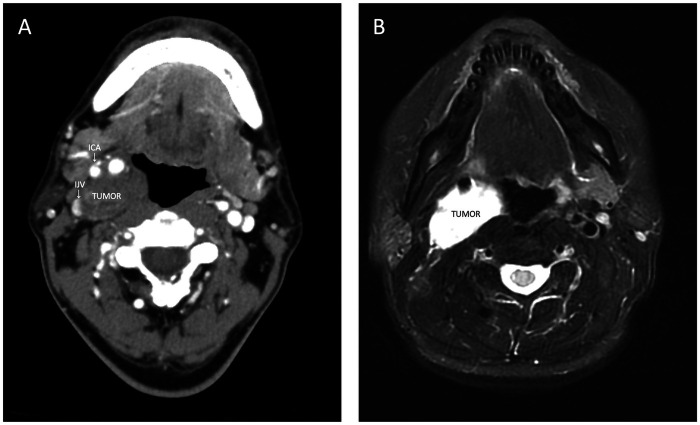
Non-surgically managed post-styloid parapharyngeal space tumor with a presumed neurogenic origin. (A) Axial contrast-enhanced CT image shows a well-demarcated enhancing mass in the right post-styloid parapharyngeal space, splaying the internal carotid artery (ICA) and internal jugular vein (IJV), with anteromedial displacement of the ICA. (B) Axial fat-saturated T2-weighted MR image shows a well-circumscribed post-styloid mass. Although the tumor location and vessel displacement pattern strongly favored a neurogenic lesion, a minor or ectopic salivary gland tumor could not be definitively excluded and remained a radiologic differential consideration. The patient was managed with active surveillance, and follow-up imaging demonstrated no interval growth over 32 months.
